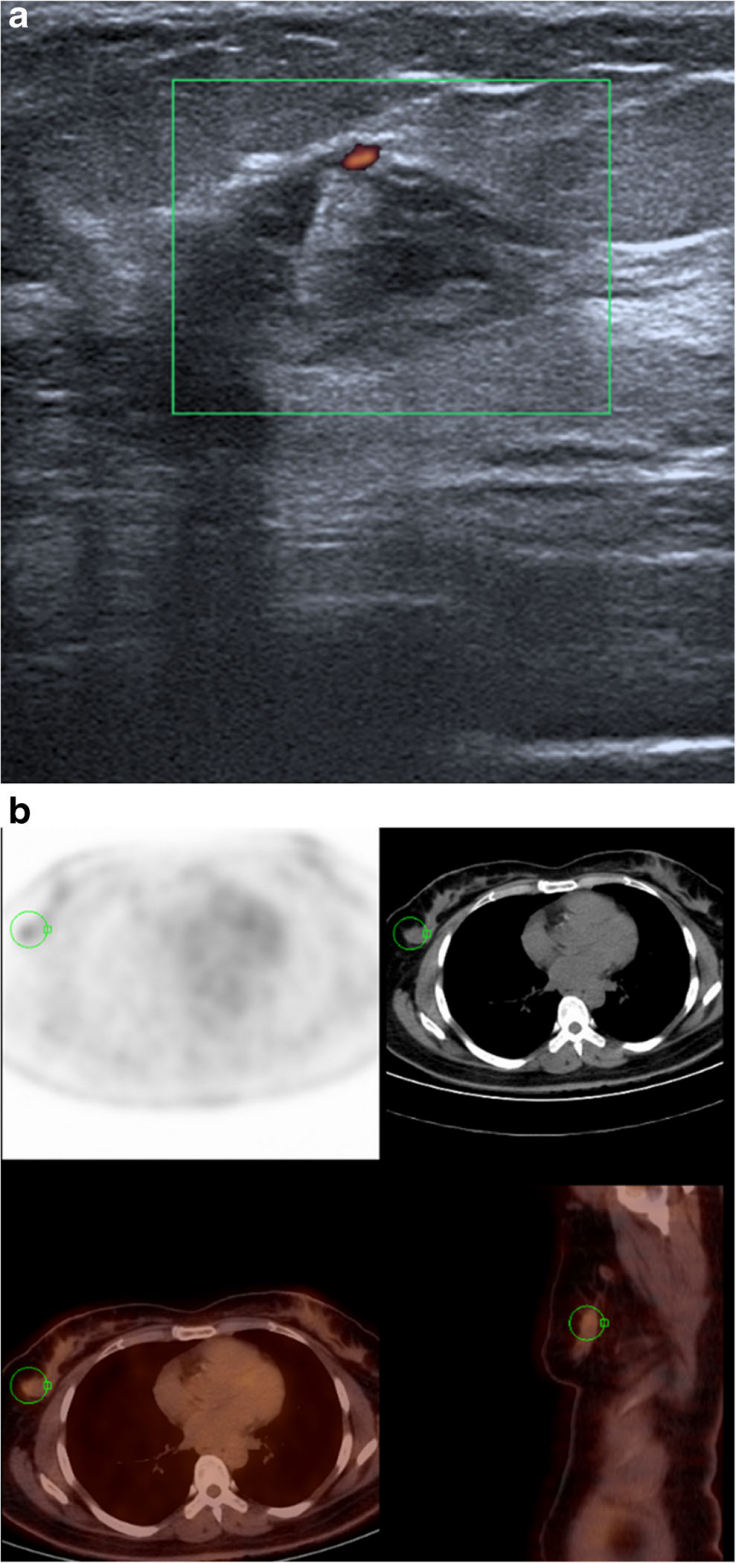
Lymphoma. a Ultrasound of the right breast showing a poorly demarcated, mixed echogenicity mass, with blood flow on Doppler ultrasound. b PET-CT scan in this patient shows a hypermetabolic lesion in the right breast. Microbiopsy revealed diffuse large B-cell lymphoma
